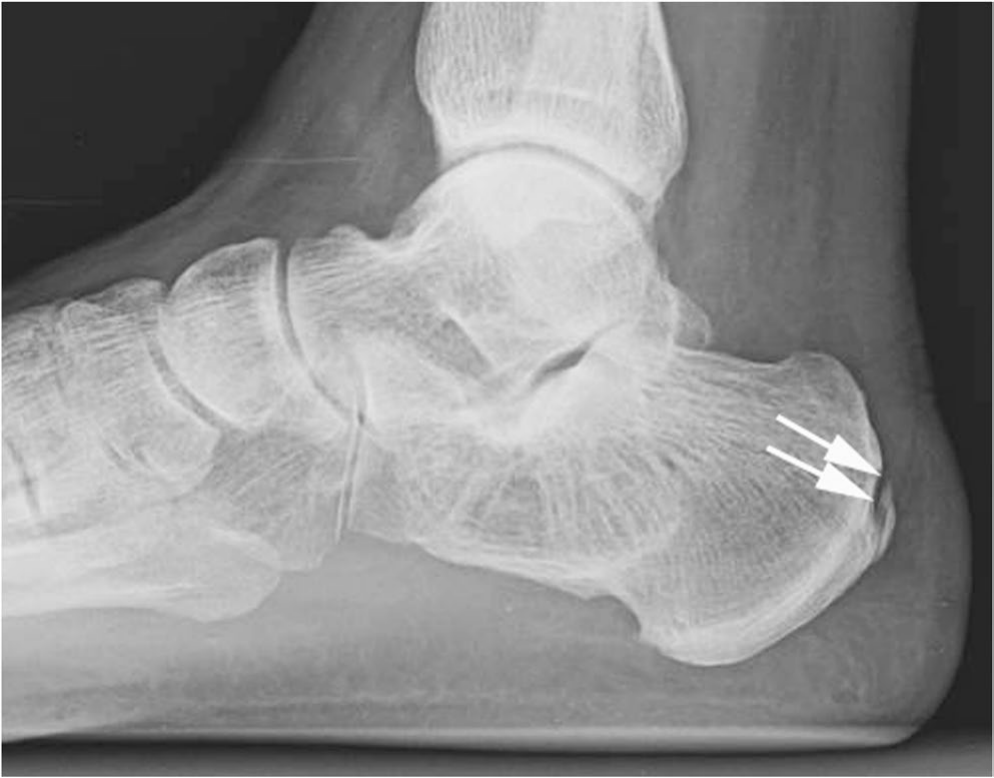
Type III calcaneal tuberosity fracture. 44-year-old man with pain after a fall. Lateral radiograph shows a mildly displaced avulsion fracture of the calcaneal tuberosity (arrow). The patient was treated conservatively, and no further imaging was obtained
